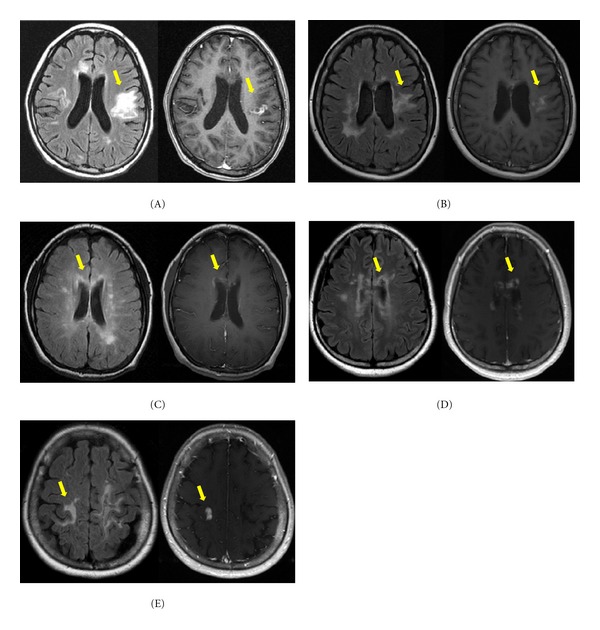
Paired fluid-attenuated inversion recovery (FLAIR) (left) and corresponding gadolinium-enhanced (right) axial images of patients who showed enhancement. Enhancement observed in extensive hemispheric lesions (A, B, and E) and periependymal lesions surrounding the lateral ventricles (C, D). The lesions reveal multiple patchy enhancement patterns with blurred margins, showing typical “cloud-like enhancement”. One exceptional lesion reveals well-marginated nodular enhancement at an extensive hemispheric lesion (E) [28].
